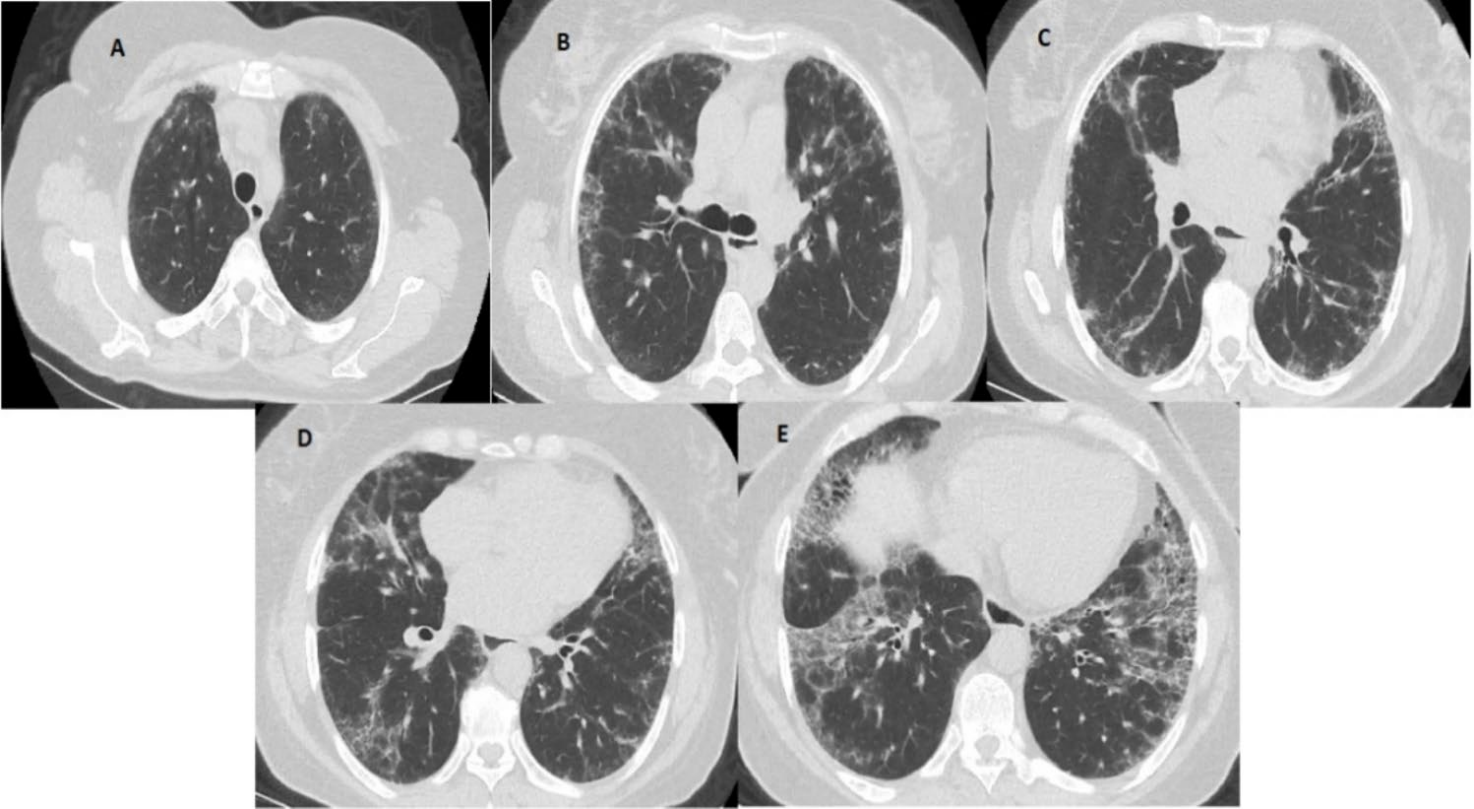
An example of a semiquantitative HRCT assessment. Five levels of HRCT images. A Origin of great vessels (ILD score 5% and MCRD score 2). B Main carina (ILD score 15% and MCRD score 2). C Pulmonary venous confluence (ILD score of 30% and MCRD score of 2). D Halfway between the third and fifth sections (ILD score 45% and MCRD score 2). E Immediately above the right hemidiaphragm (ILD score of 65% and MCRD score of 3)
